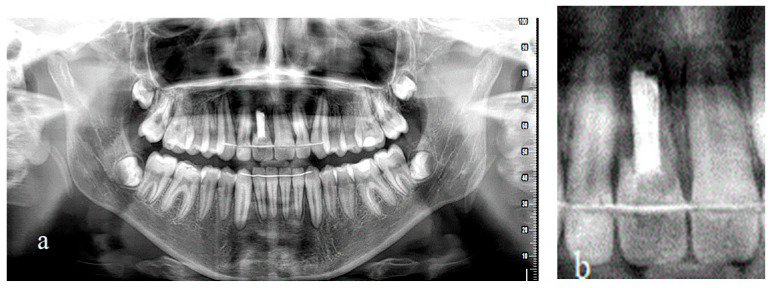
(a) Final panoramic radiograph. Fixed retainers for upper and lower arch. Bone healing around 11 was completed. (b) Magnified detail from the adjacent panoramic radiograph, presenting severe root resorption of 11 and unfortunately apical root resorption of 12 and 21.
